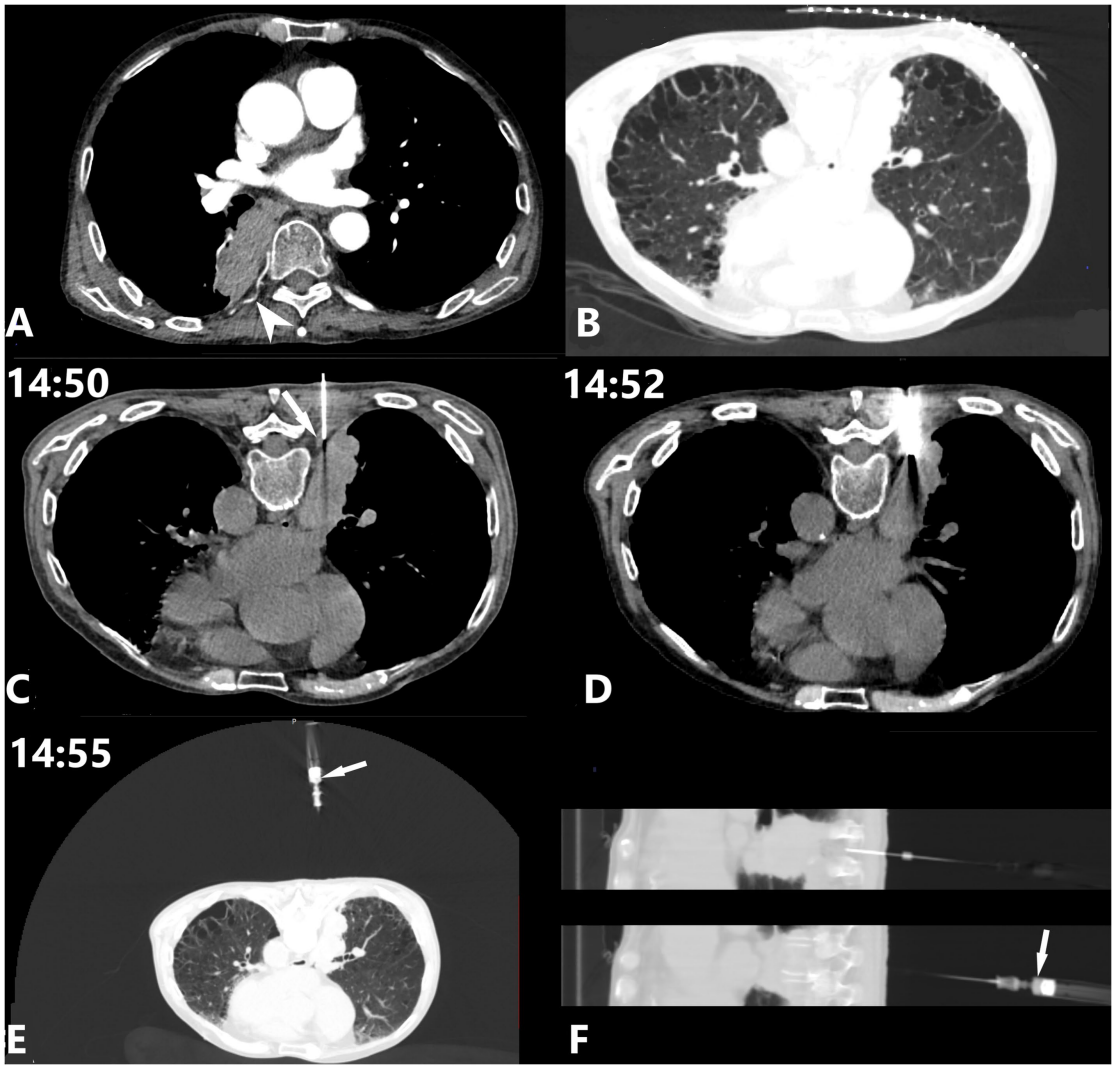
A 71-year-old male with a 10+ year history of recurrent cough and sputum production was admitted due to worsening symptoms and chest pain for 3 days. (A,B) Chest CT scan demonstrates emphysema with multiple bullae in both lungs, and an oval soft tissue density mass (approximately 3.0 × 6.0 cm) in the right lower lobe adjacent to the spine. An ICA (white arrowhead) courses along the posterior margin of the right lung mass. (C) To minimize the risk of pneumothorax, the puncture path was planned to avoid areas of emphysema and lung bullae. The coaxial puncture needle reaches the extrapleural pleura, with its medial edge adjacent to the origin segment of the ICA (white arrow). (D) Four tissue samples were obtained, each measuring 2.2 cm in length. (E,F) Bright red blood rapidly spurted into the 5 mL syringe (white arrow), with an approximate volume of 0.5 mL.
